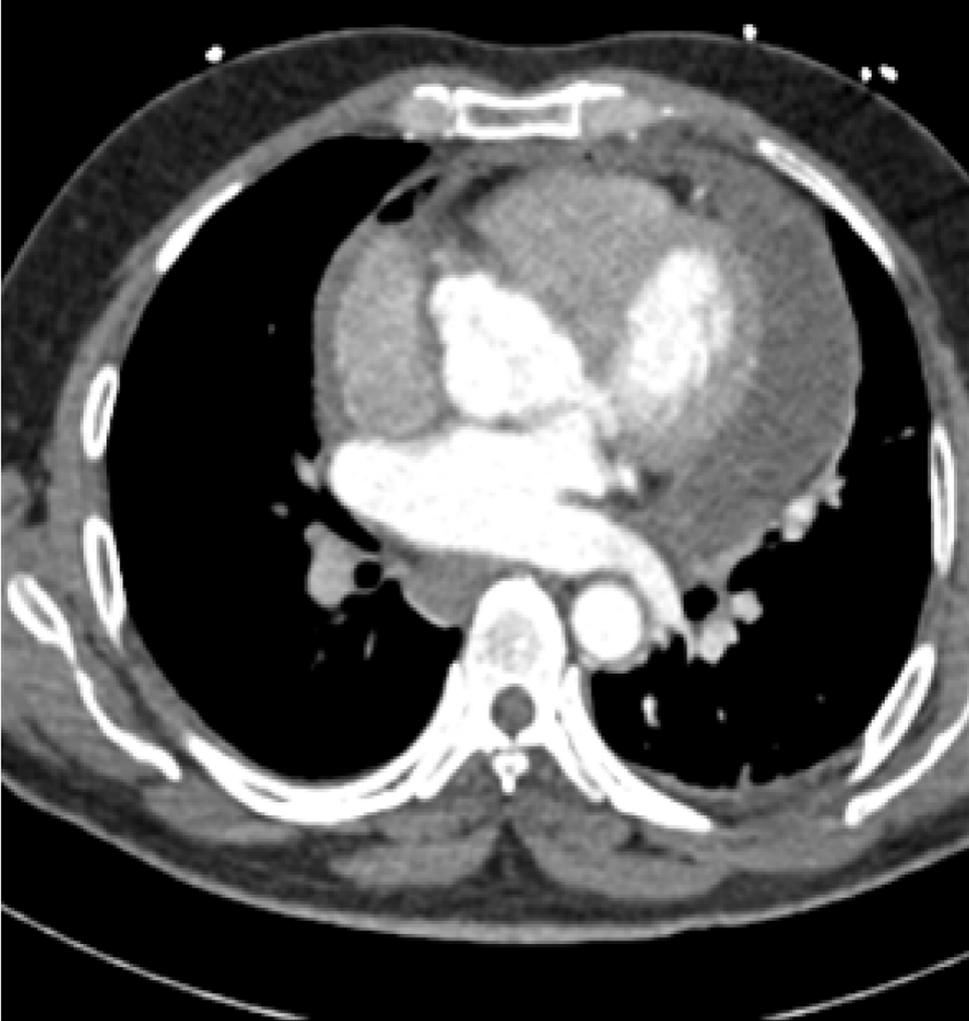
CT Scan Image of the Aortic Sinus that Showed Dilated Distorted RCS
